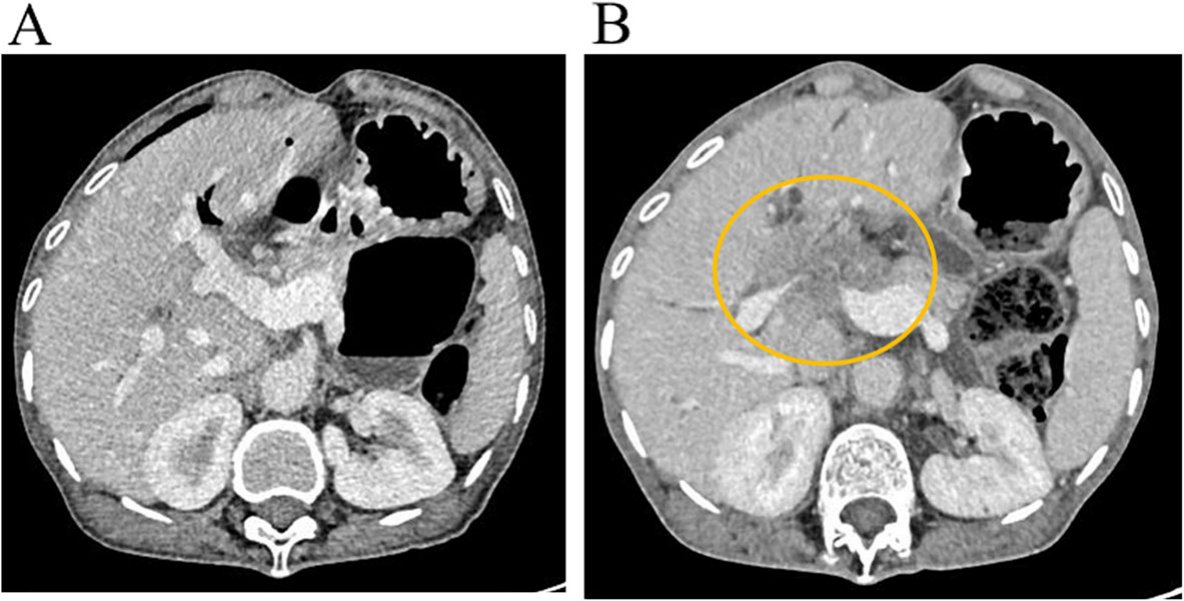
a Computed tomography was performed at a regular checkup. The scan shows no gallstones or tumor formation in the liver at 62 years and 10 months of age. b A liver tumor at the porta hepatis is revealed by computed tomography. The patient was aged 63 years and 6 months. Yellow circle: the tumor at the porta hepatis
